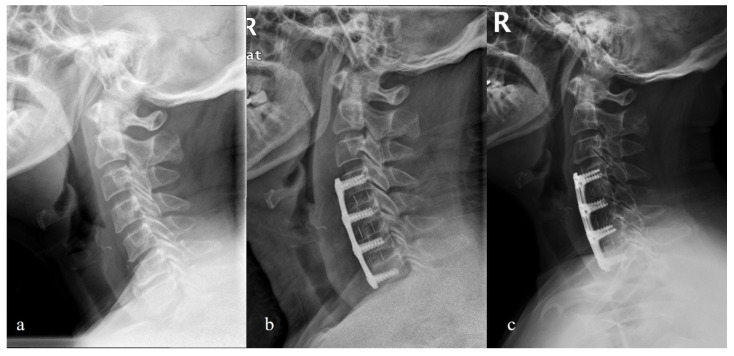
Lateral radiographs of one patient receiving anterior plate and cage system. (a) Preoperative C2-C7 Cobb angle was 10 degrees; (b) postoperative C2-C7 Cobb angle increased to 22 degrees; (c) the C2-C7 Cobb angle maintained 18 degrees at the 3-year follow-up.
